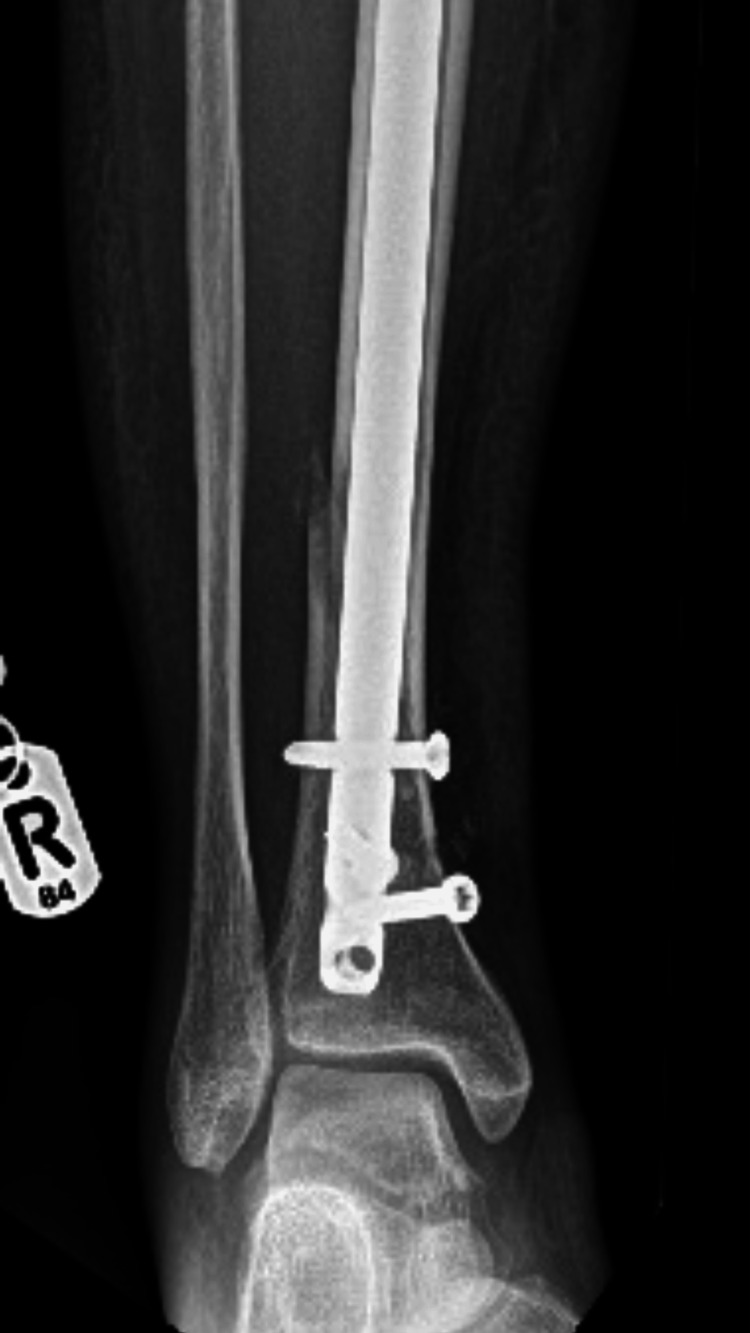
A postoperative X-ray from the anterior-posterior (AP) view.
